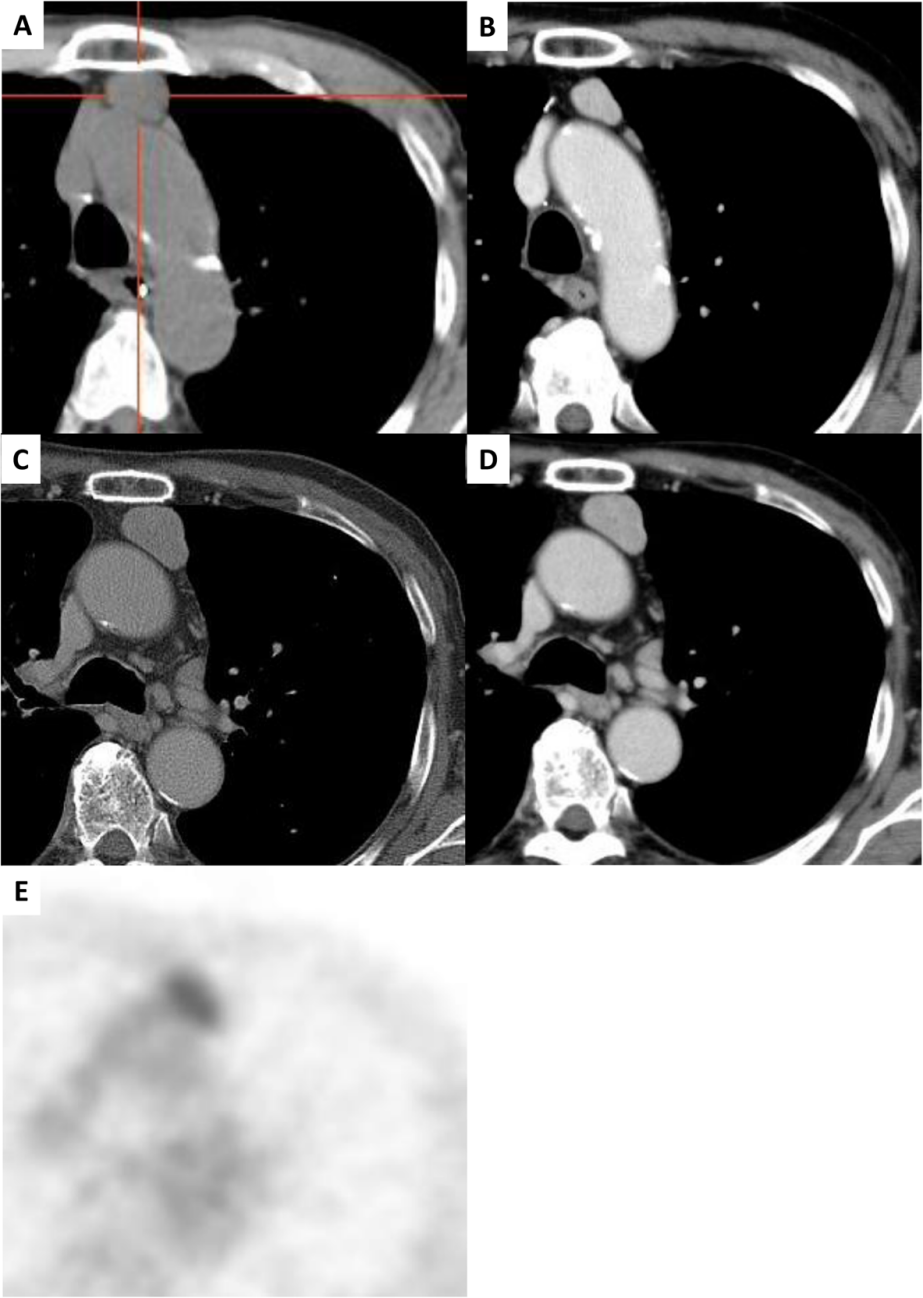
Anterior mediastinal mass. Chest computed tomography (CT) revealed a 2.5-cm well-defined homogenous mass in the anterior mediastinum at the time of diagnosis with autoimmune pancreatitis (a), and the tumor decreased in size to 2 cm on CT with oral steroid treatment 6 years ago (b). The tumor had regrown to 3 cm at the time of referral to our department on CT (c, d), and positron emission tomography showed a high maximum standardized uptake of 3.6 by the anterior mediastinal mass lesion (e)
